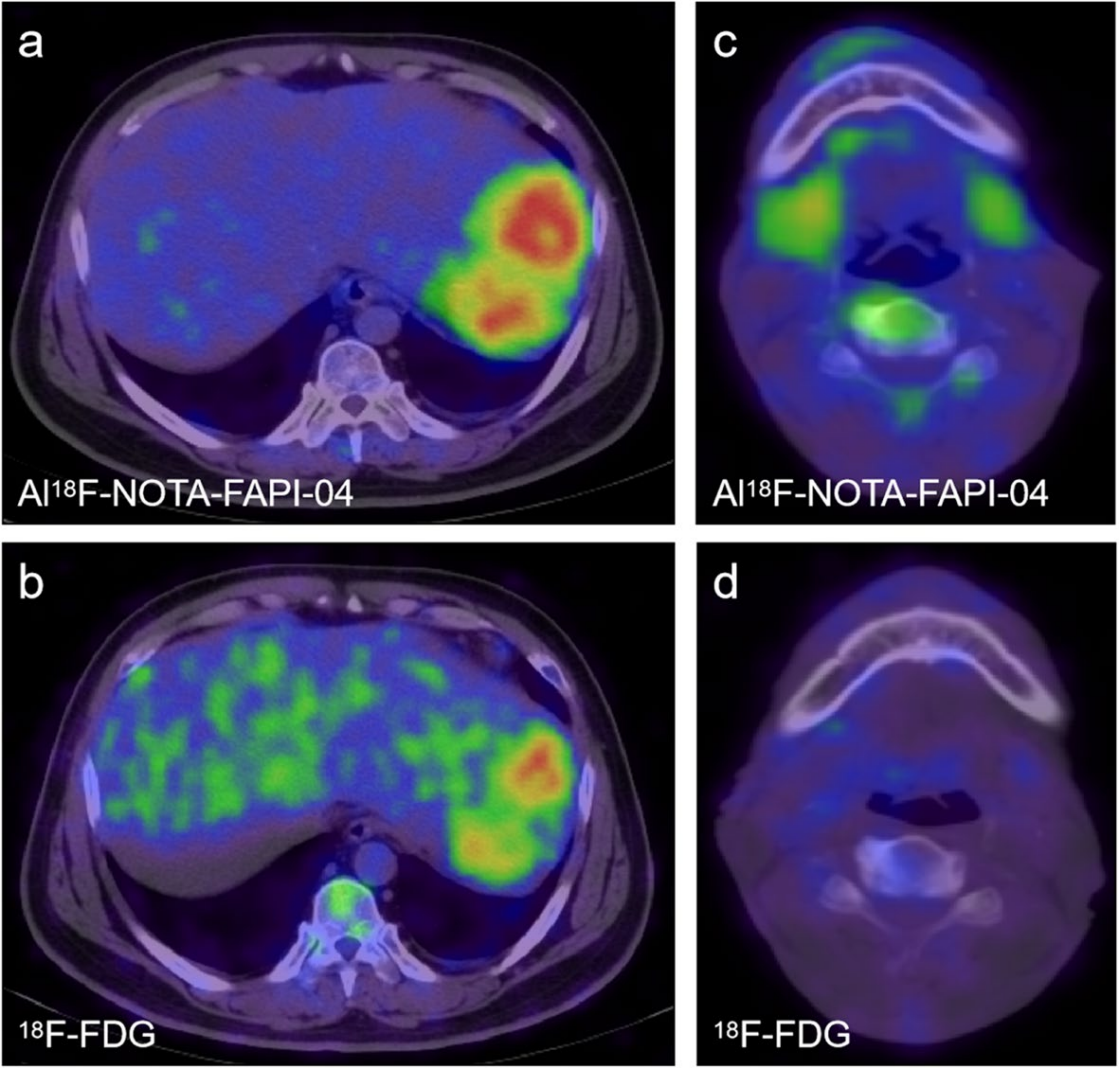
Comparisons between Detection of Extra Abnormal Al^18^F-NOTA-FAPI-04 and ^18^F-FDG Uptake. a and b both showed Al^18^F-NOTA-FAPI-04 and ^18^F-FDG uptake in the liver metastases (SUV_max_: 10.6 vs. 6.0), demonstrating a better TBR of FAPI than FDG. c showed Al^18^F-NOTA-FAPI-04 uptake in cervical bone hyperplasia, while d showed no ^18^F-FDG uptake (SUVmax: 3.2 vs. 1.7), suggesting that skeletal lesions should be carefully analyzed
